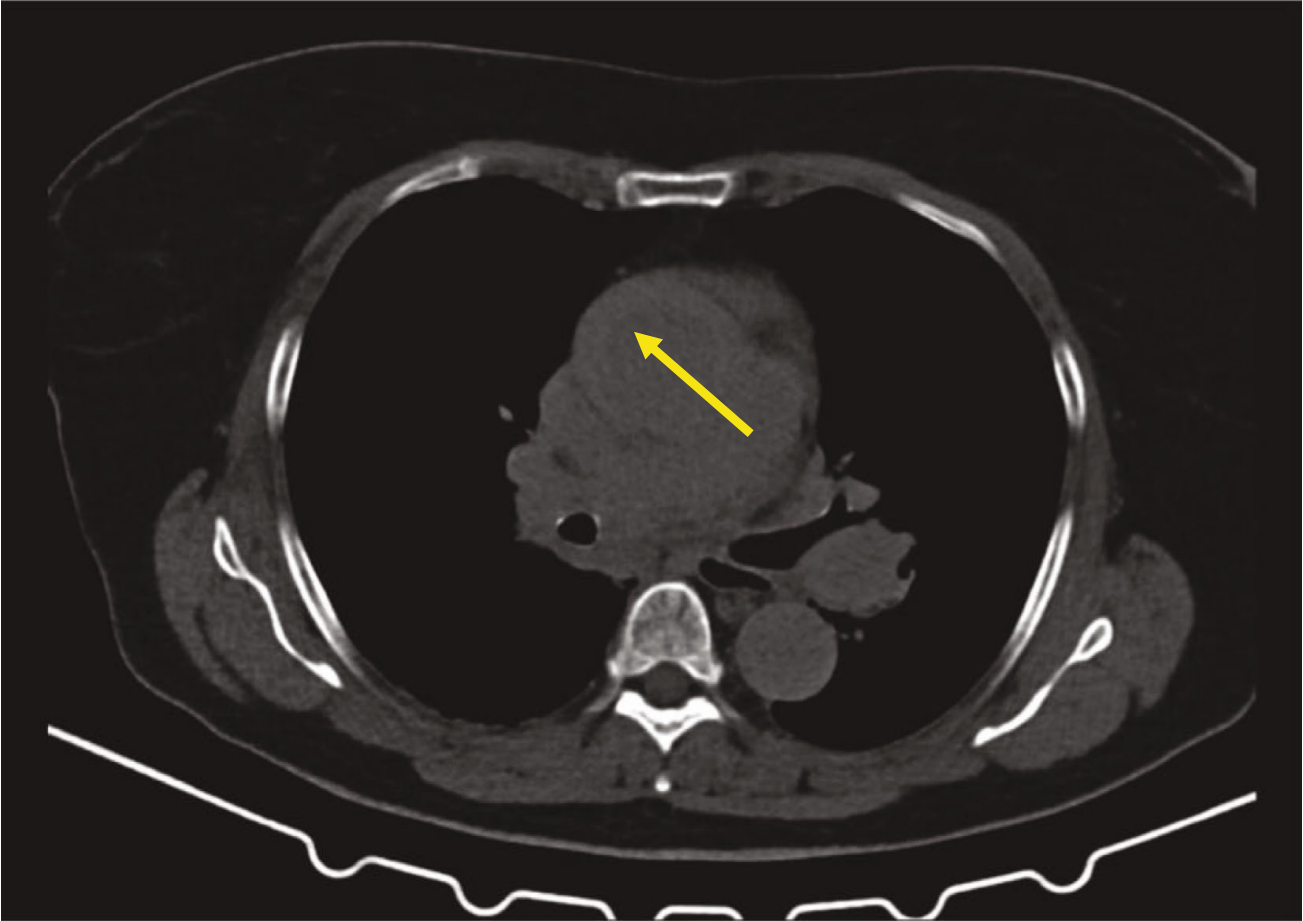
Plain computed tomography of the aorta performed at the referral hospital showed a focal, crescent-shaped, high-attenuation region with eccentric thickening of the aortic wall (indicated by an arrow), with a total diameter of approximately 48 mm.
